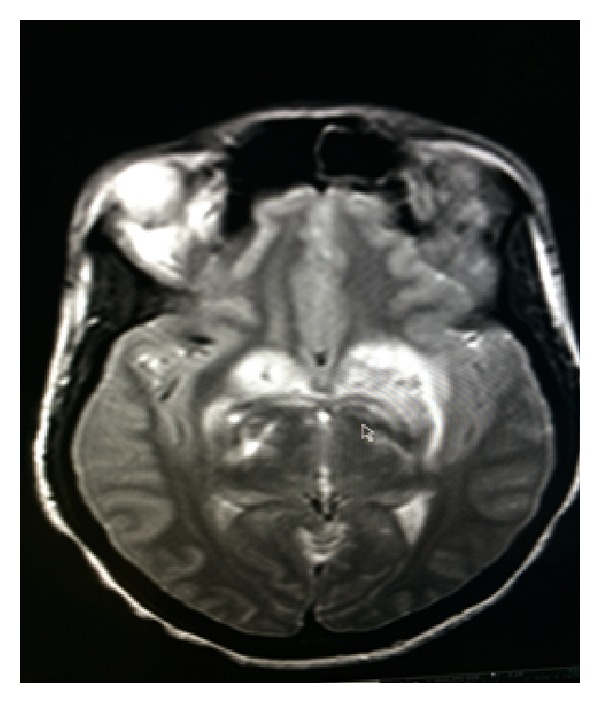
MRI-T2-Axial View: vasogenic edema of basal ganglia.
